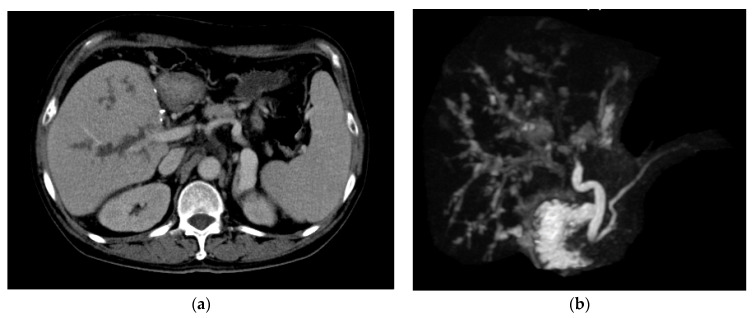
A representative of imaging study in AMR with ischemic biliary stricture. In this representative, (a) CT shows dilated biliary tract with irregular lining. (b) Magnetic resonance cholangiopancreatography shows multiple saccular dilatations of the biliary tract.
